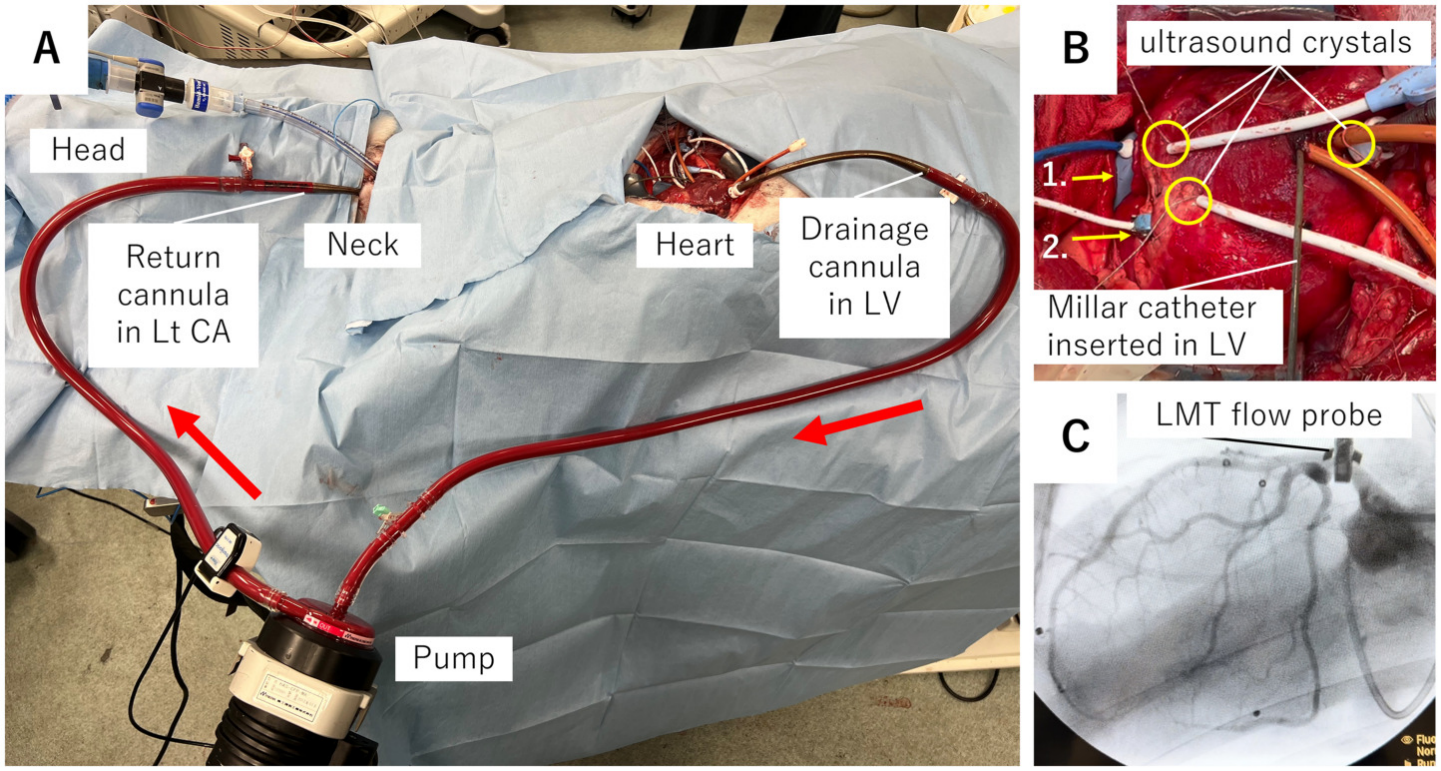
Overview of experimental setup. (A) Picture describing LVAD system. Blood sucked from a drainage cannula at the left ventricle flows through a pump to the carotid artery via the return cannula. Red arrows show the direction of blood flow. (B) Picture showing the location of ultrasound crystals and flow probe. (1) A flow probe at pulmonary artery; (2) a flow probe at left main trunk of coronary artery. (C) Cine film showing the left coronary arteries and the flow probe at the left main trunk. CA, carotid artery; LMT, left main trunk; Lt, left; LVAD, left ventricular assist device.
